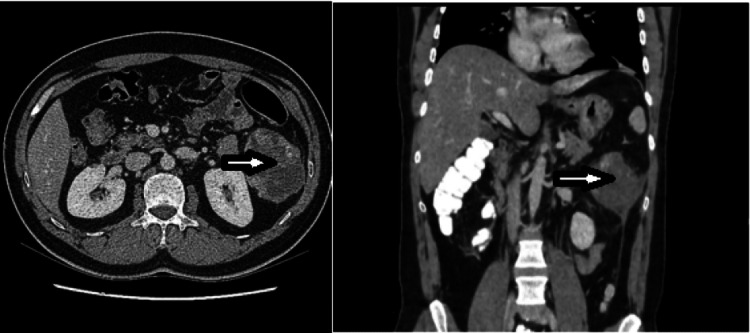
CT images CT abdomen and pelvis show a non-enhancing mass cystic and solid components abutting the kidney and a hypo-dense center (arrow).
